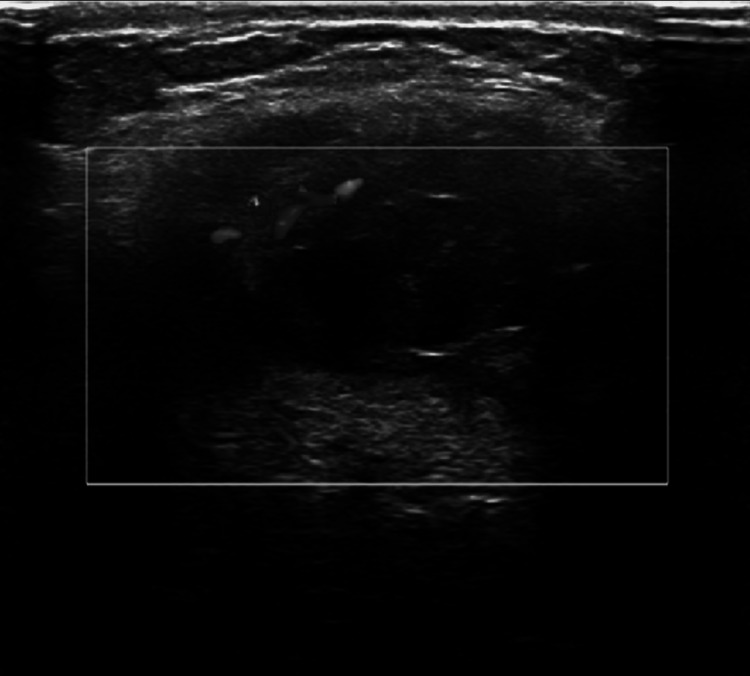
Ultrasonography of neck mass (transverse Doppler view).
